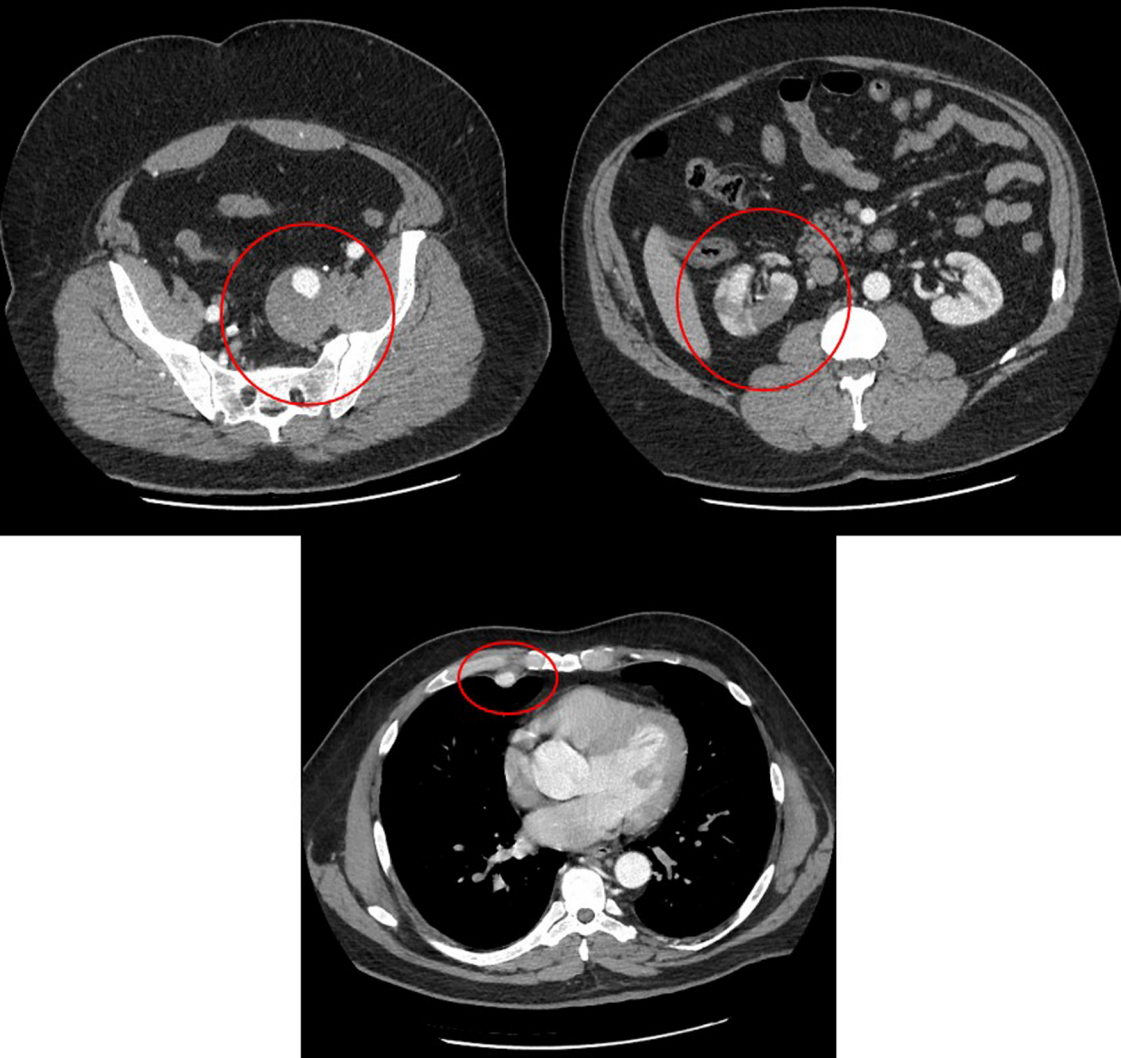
Computed tomography angiogram showing right renal infarction (right panel), left internal iliac artery aneurysm (left panel), and right internal mammary artery aneurysm (bottom panel).
